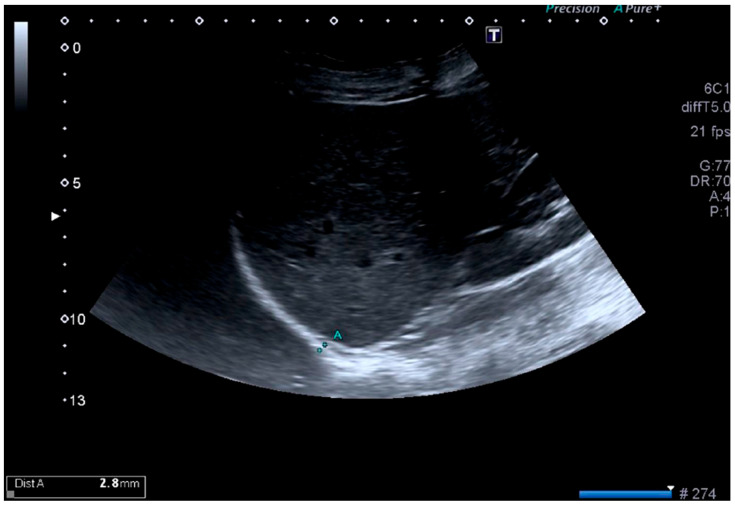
Ultrasonographic measurement of diaphragmatic thickness. Representative B-mode ultrasound image showing diaphragmatic thickness measurement at the zone of apposition. The diaphragm is visualized as a hypoechoic muscle layer between the echogenic pleural and peritoneal lines.
